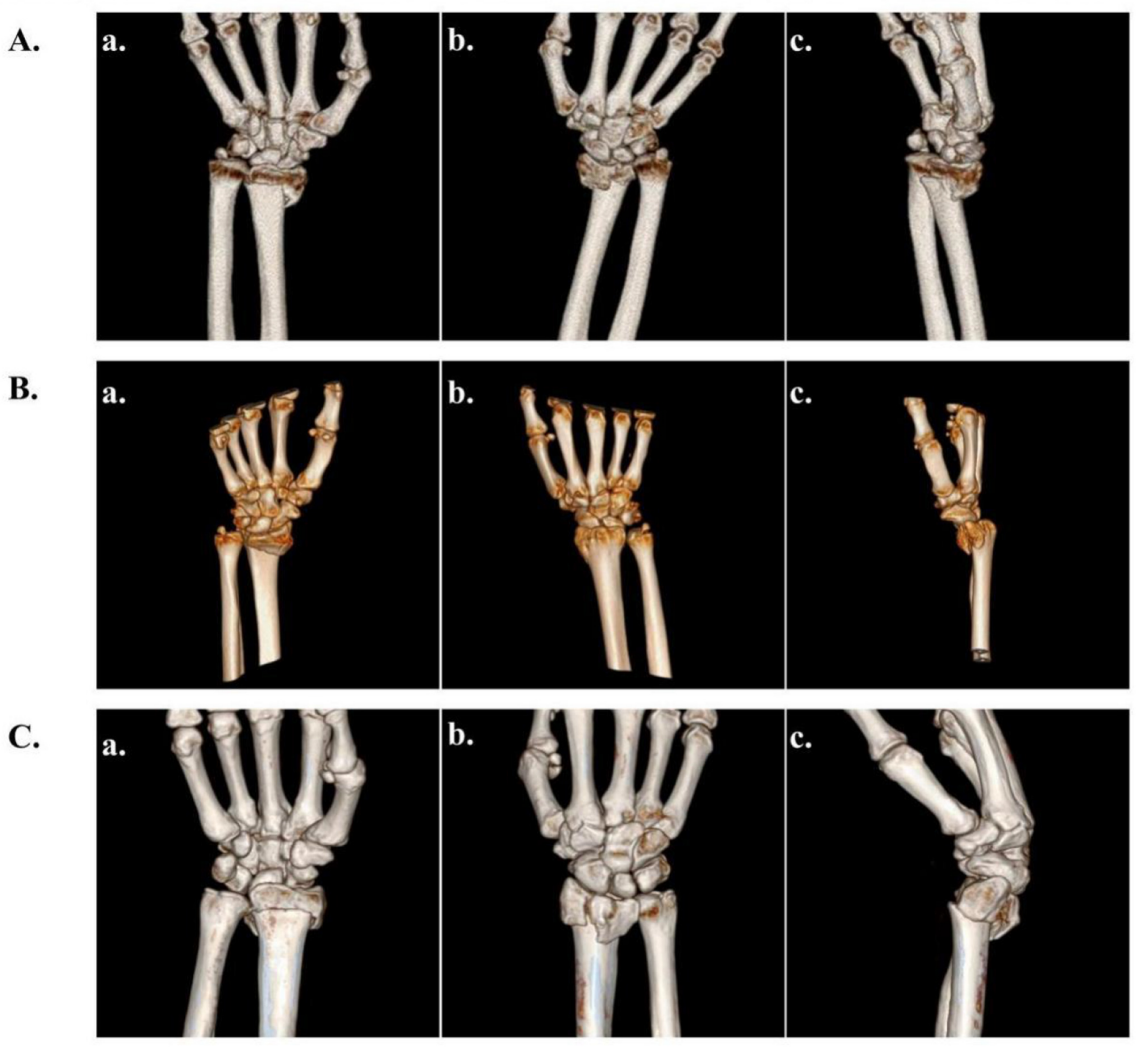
AO typing of distal radius fractures. (A) Frontal and lateral 3D reconstruction of CT images of extra-articular fractures (Type A). (B) Frontal and lateral 3D reconstruction of CT images of partial intra-articular fractures (Type B). (C) Frontal and lateral 3D reconstruction of CT images of complete intra-articular fractures (Type C).
